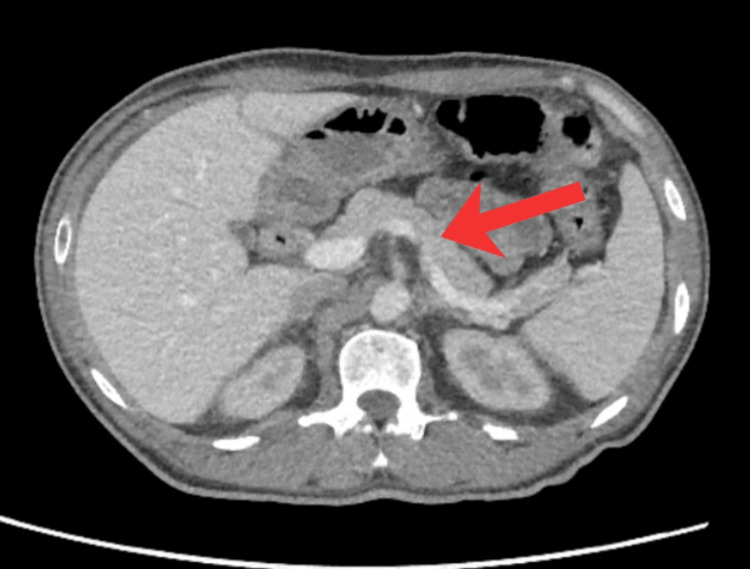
Contrast-enhanced CT of the abdomen demonstrating a normal-appearing pancreas without enlargement, peripancreatic fat stranding, fluid collections, or necrosis, despite serum lipase levels exceeding 4,000 U/L
